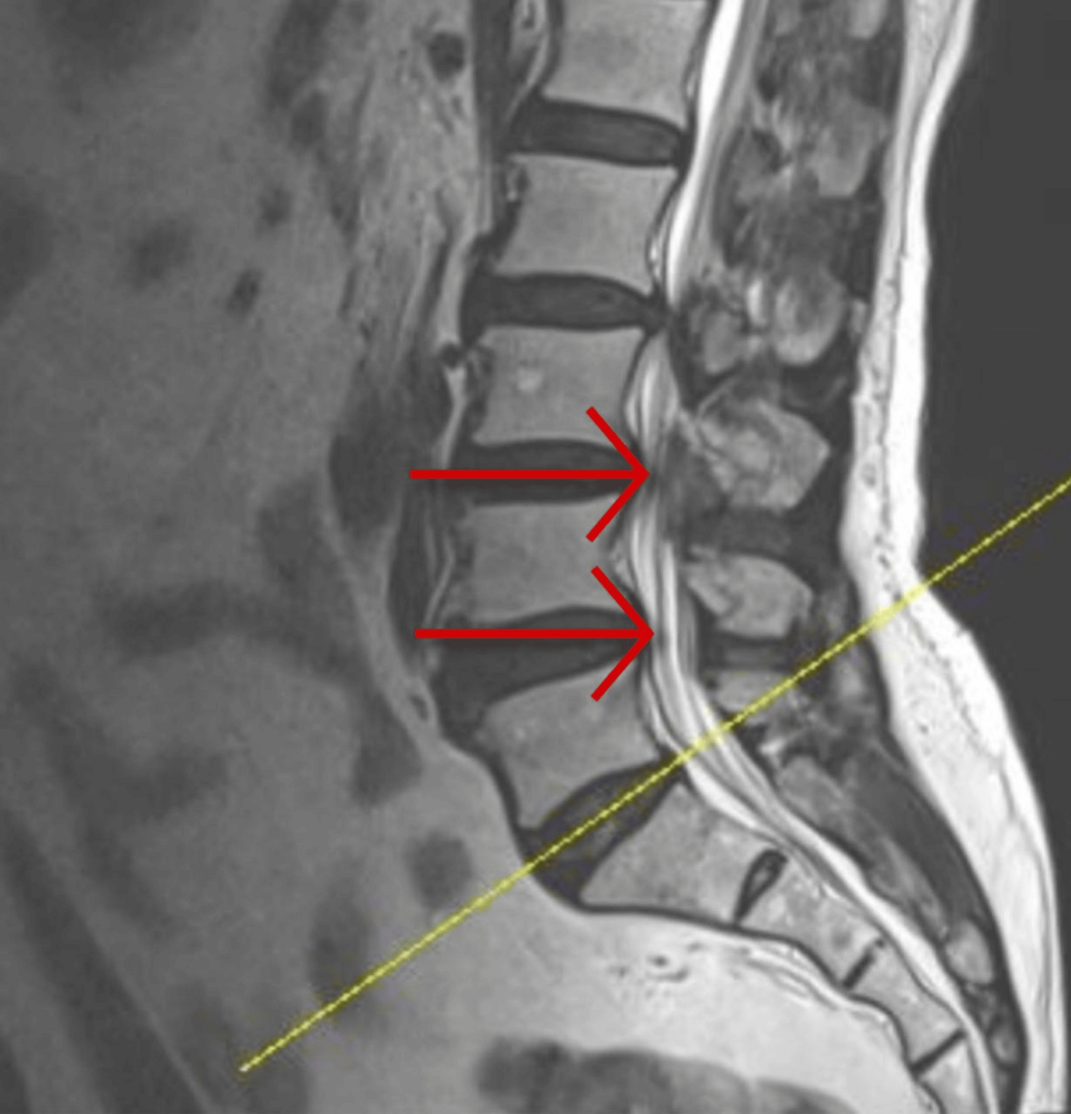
Magnetic resonance imaging of lumbar spine Asymmetric diffuse disc bulge at the L4/L5 level, resulting in mild spinal stenosis.
